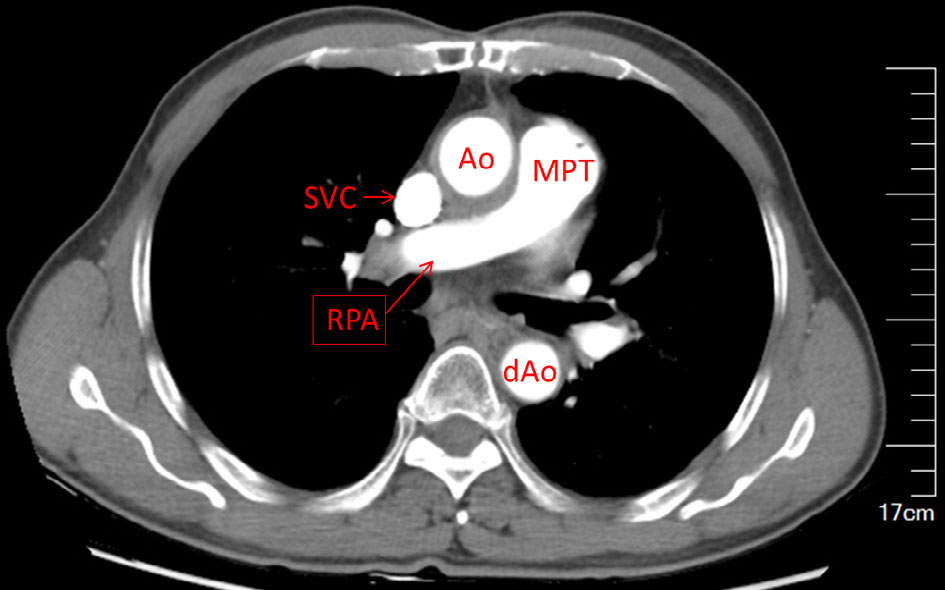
Thoracic CT scan showing no recurrence at 69 months after surgery.
